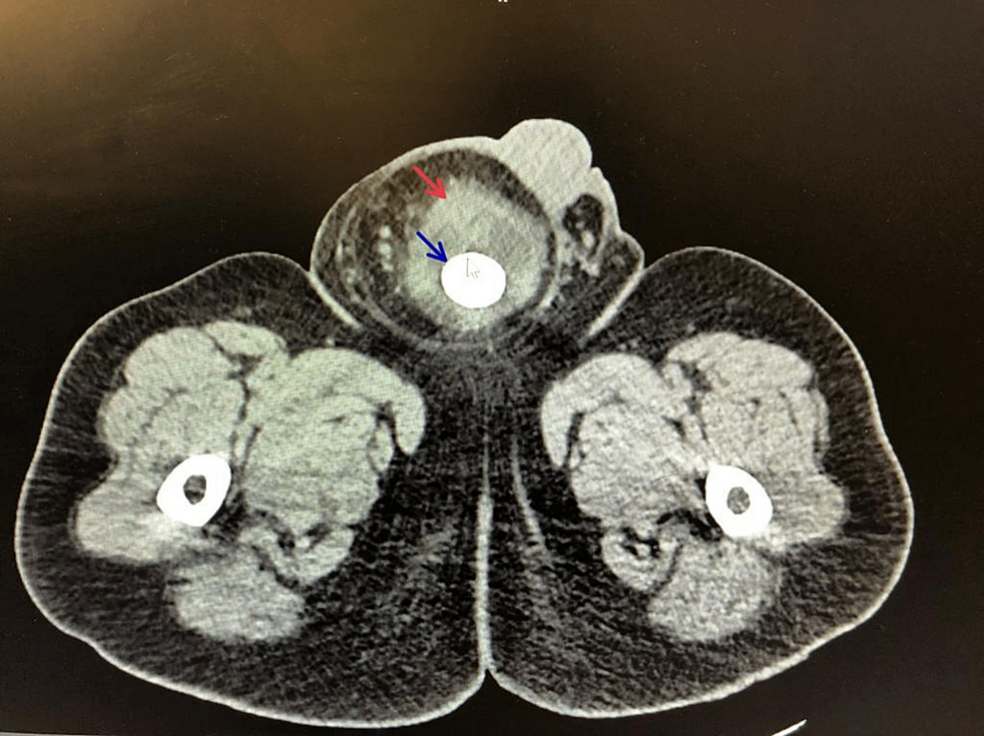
A axial CT scan showing a pantaloon urinary bladder herniation (red arrow) with a calculus (blue arrow).
